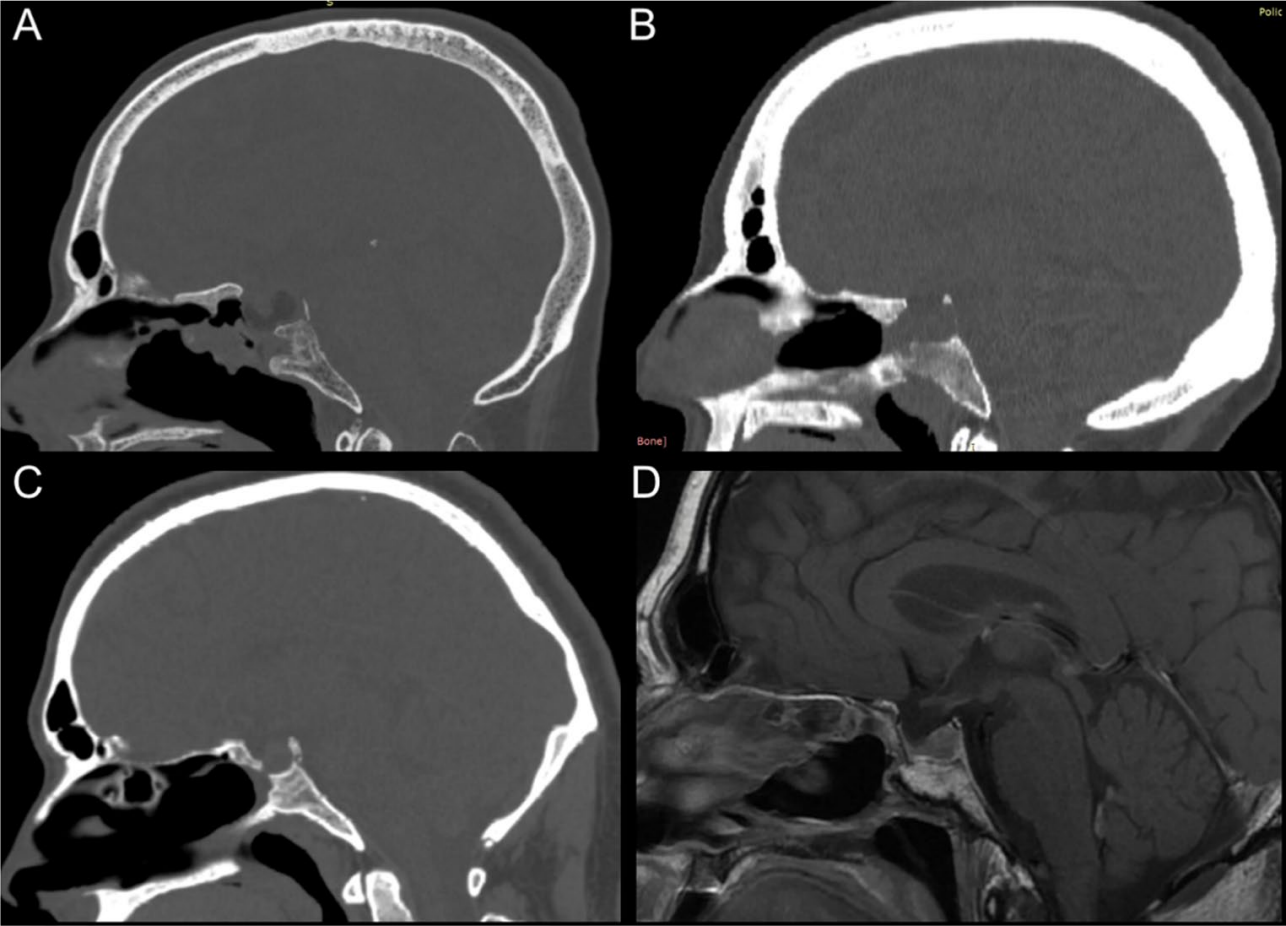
Radiological evaluation of osteointegration. Medpor is usually well recognized in MR imaging (D) as hypointense in T1- and T2 weighted images, while it is less evident in CT images, being hypo- or isodense. A, B: CT scans performed respectively 7 and 3 years after EEA with Medpor and nasoseptal: no osteointegration was identified. C; CT scan documenting partial osteointegration of Medpor 4 years after surgery. D: Brain MRI performed 7 years after endoscopic transnasal surgery with septal flap positioned: Medpor appears well osteointegrated, as confirmed intraoperatively
